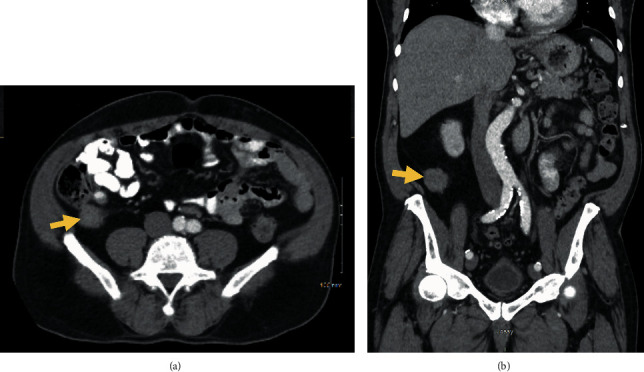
(a) Axial abdomen/pelvis CT showing a soft tissue mass (arrow) at the tip of the appendix. (b) Coronal image of the soft tissue mass (arrow) at the tip of the appendix.
